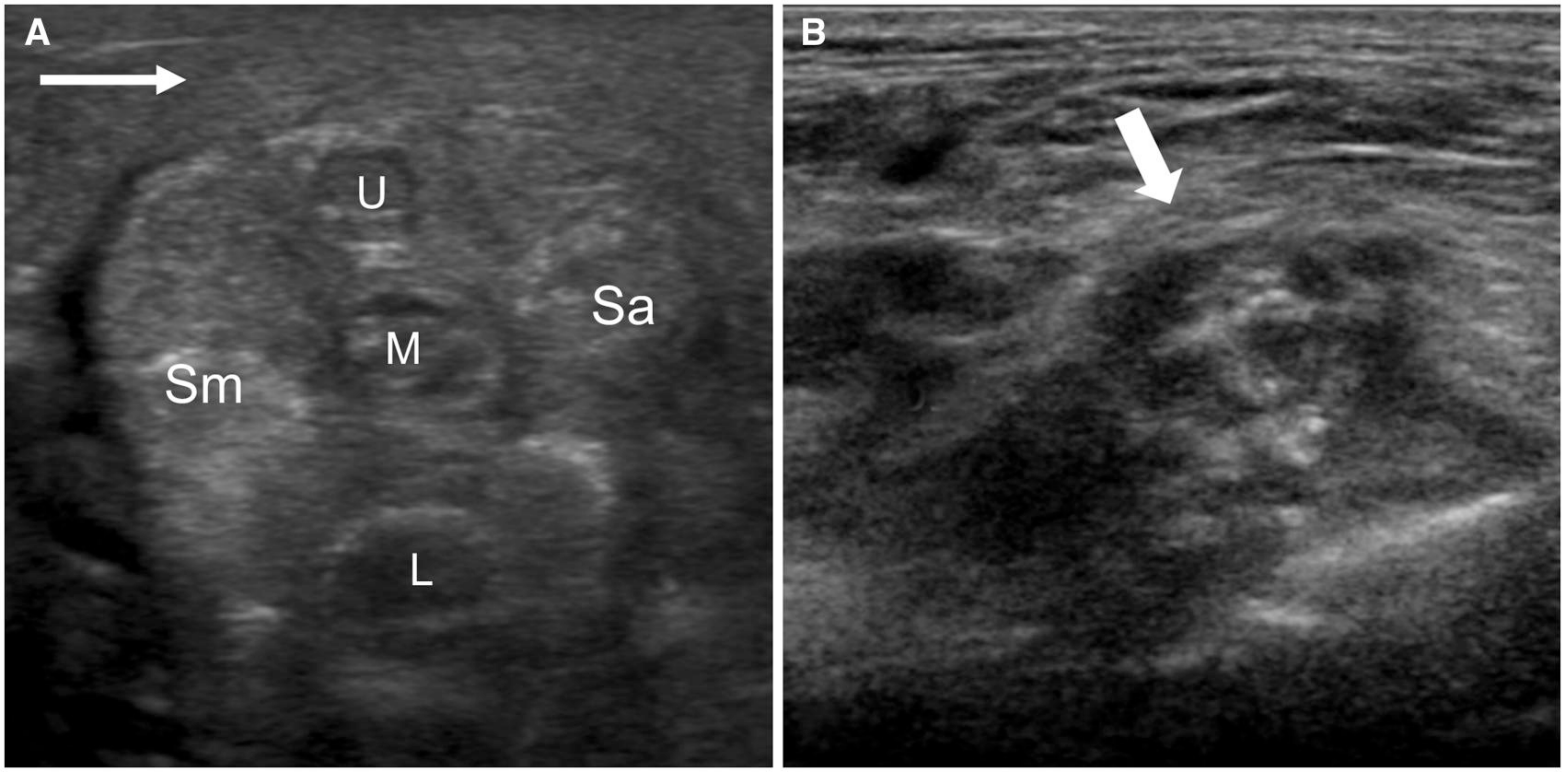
(A) Transverse ultrasound showing severe irradiation plexopathy of the interscalene triangle with perineural fat fibrosis leading to poor definition of the trunks (arrows) as well as severe dermal and subcutaneous thickening and hypoechogenicity due to fibrosis. (B) Transverse ultrasound showing moderate to severe irradiation plexopathy of the divisions, which are thickened, ill-defined, and clumped with hypoechogenicity of the adjacent fat due to fibrosis (arrows). Abbreviations: Sa = scalenus anterior muscle; Sm = scalenus medius muscle.
